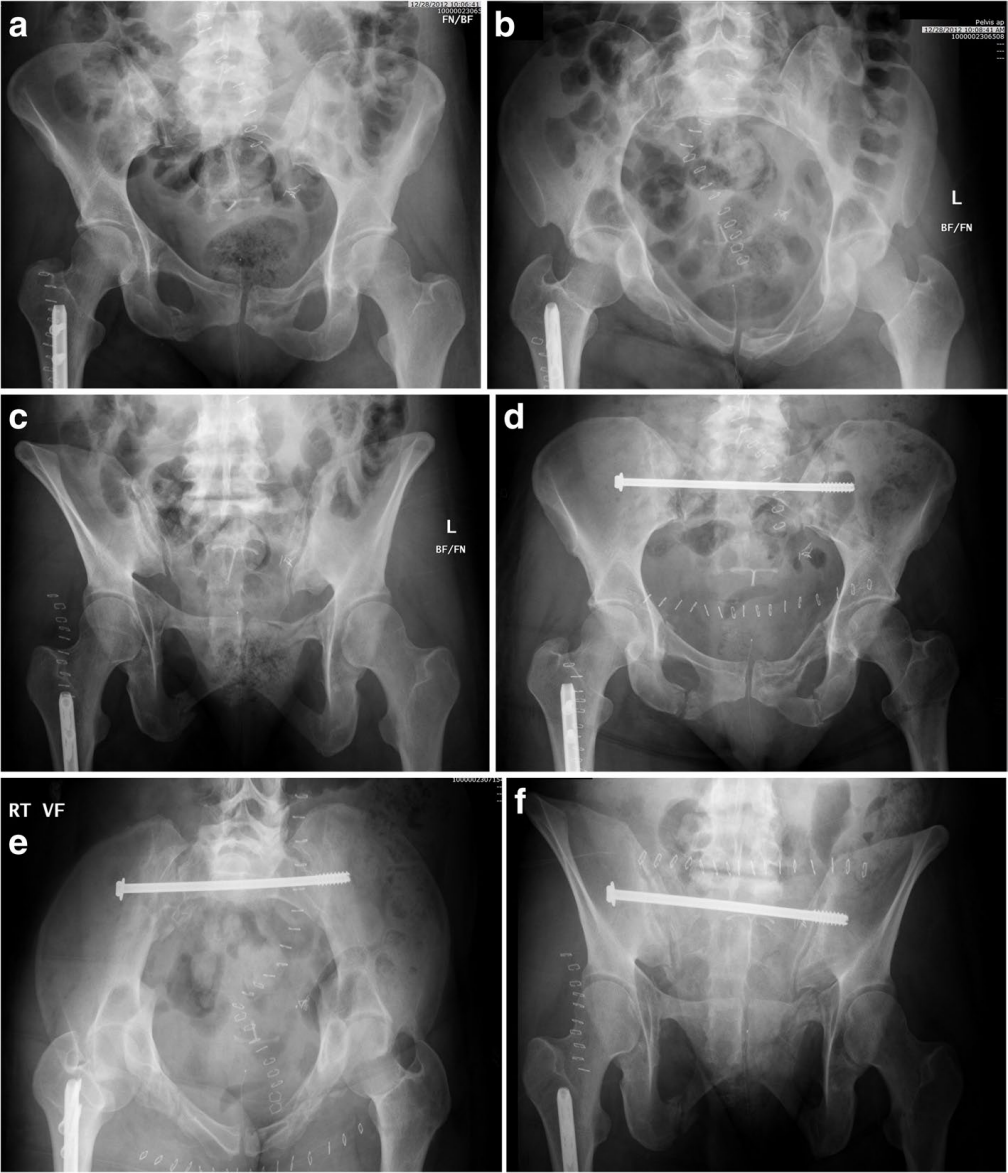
Radiographic pelvic images demonstrating a right SI fracture-dislocation (A-C) reduced and fixed with a trans-sacral screw (E-F)
